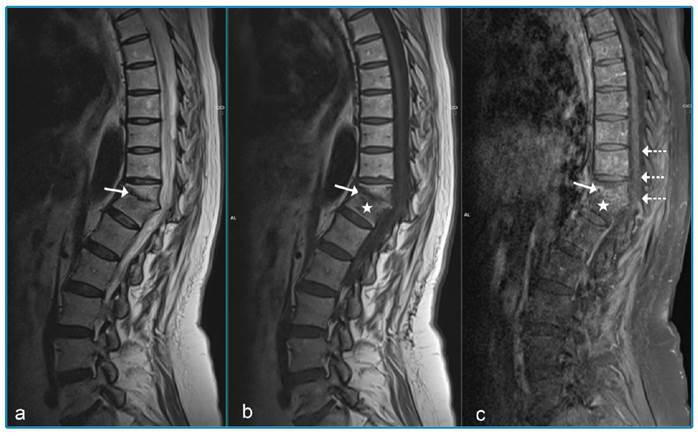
Post-treatment MRI. T2 W (a): loss of T11-T12 intervertebral disc space and compression (arrow) in T11 vertebral body is observed. T1 WI (b): signal losses in the vertebral bodies and disc region seem to have almost completely recovered. Contrast-enhanced T1 WI (c): contrast enhancement is not seen in the vertebral bodies (star) and posterior epidural space (dashed arrow), and the contrast enhancement in the vertebral plates is significantly reduced (arrow).
